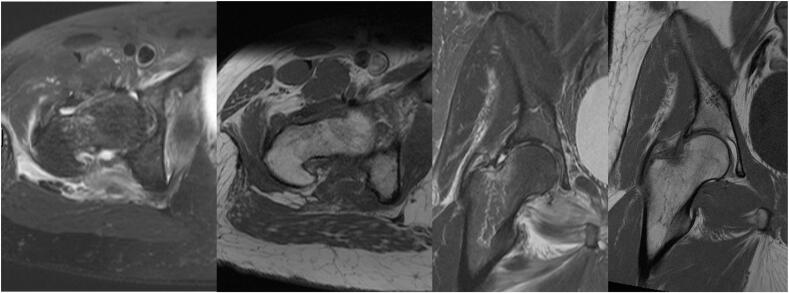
MRI of the right hip demonstrating T1 weighted (A and C) and T2 weighted (B and D) axial and coronal views, revealing an incomplete femoral neck fracture with an associated high attenuated signal representing acute inflammation.
